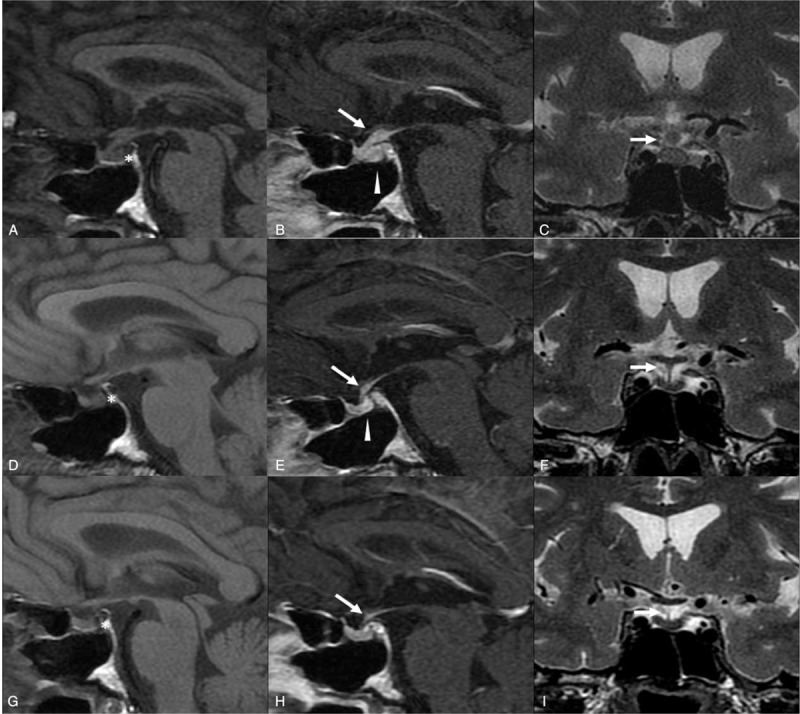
Magnetic resonance imaging (MRI) sagittal T1-weighted findings before (A, D, G) and after (B, E, H) gadolinium injection and coronal T2-weighted images (C, F, I). (A–C) Images captured in December 2013 before mycophenolate mofetil (MMF) therapy, showing loss of spontaneous T1 hyperintensity in the posterior pituitary (^∗^) associated with thickening of the pituitary stalk (white arrow). Intense enhancement of the posterior pituitary (arrow head) is evident in the pituitary stalk and hypothalamus. (D–F) MRI findings in June 2014 after 3 mo of MMF therapy, showing partial regression of the nodular thickening of the pituitary stalk (white arrow) and persistent enhancement of the posterior pituitary (arrow head). (G–I) MRI findings in January 2015 after 10 mo of MMF therapy, showing persistent loss of spontaneous T1 hyperintensity in the posterior pituitary (^∗^) and complete regression of the nodular thickening of the pituitary stalk (white arrow). The pituitary stalk exhibited normal enhancement and a normal appearance.
